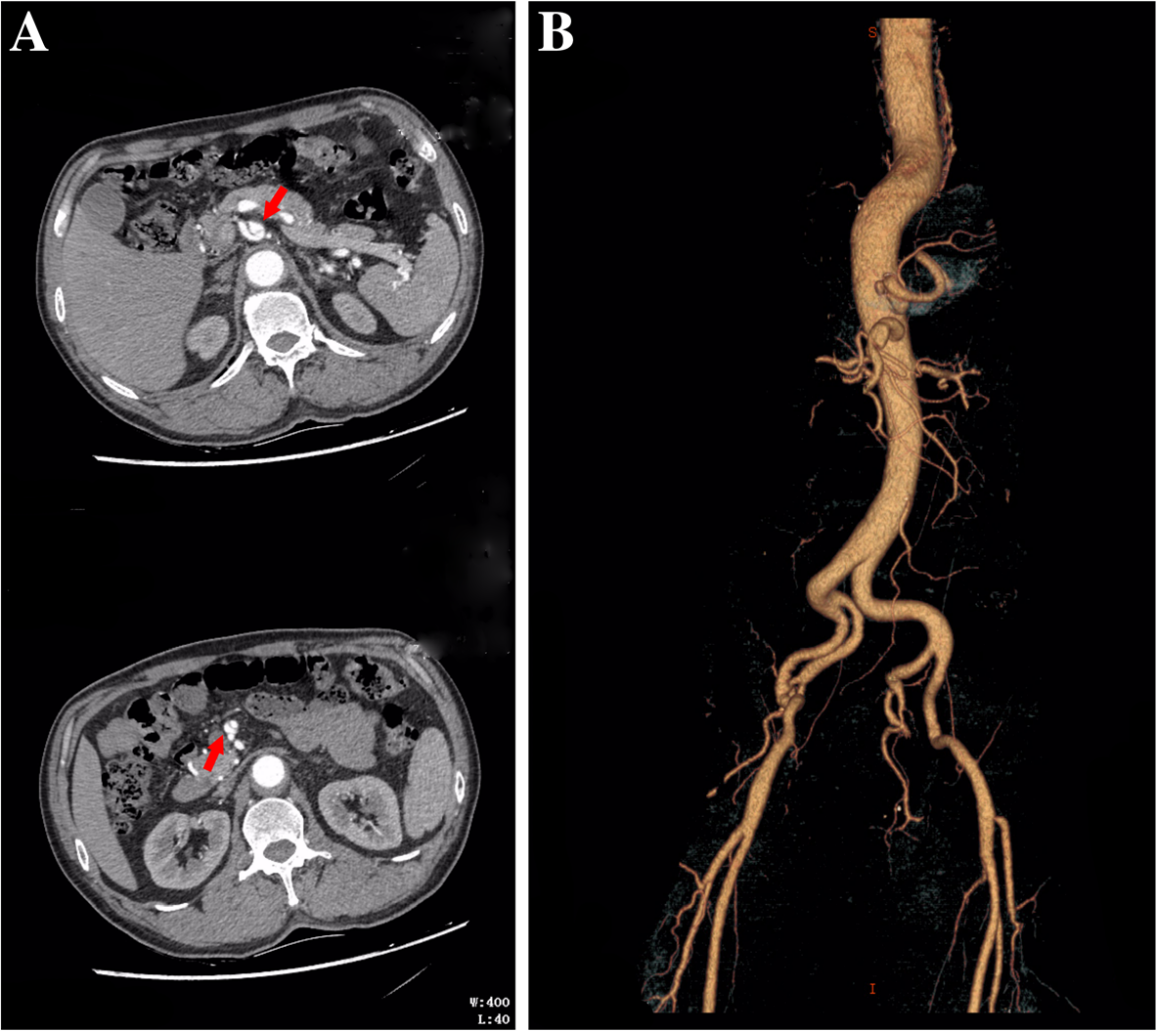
Radiographic findings of the patient. a Multi-slice computed tomography shows abdominal aortic dissection with the celiac artery and the superior mesenteric artery (Red arrow) involved. b 3D-reconstructed computed tomography angiogram shows tortuosity of abdominal and iliac aorta
